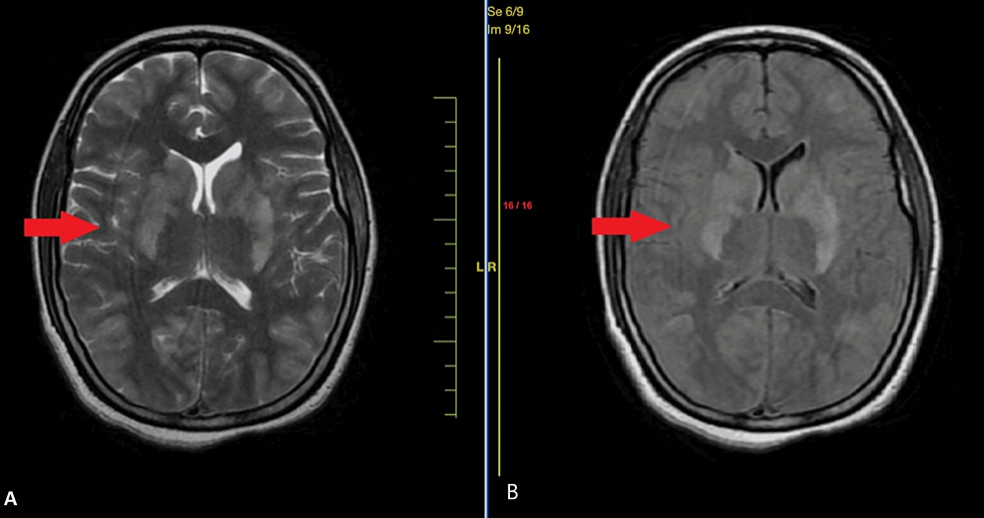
(A) High signal seen on the T2/FLAIR image in bilateral basal ganglia. (B) No restriction diffusion seen on DWI DWI: diffuse width imaging FLAIR: fluid-attenuated inversion recovery
